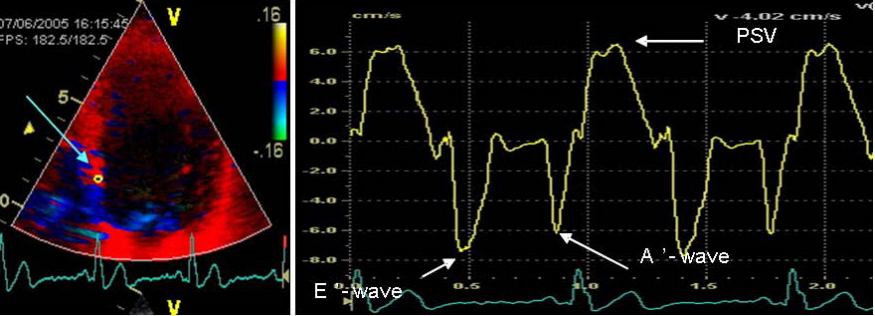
Tissue Doppler echocardiography. To the left, an apical four-chamber view picture with the sample volume placed at the basal portion of the septal wall. On the right, all the variables extracted from on-line recording.
